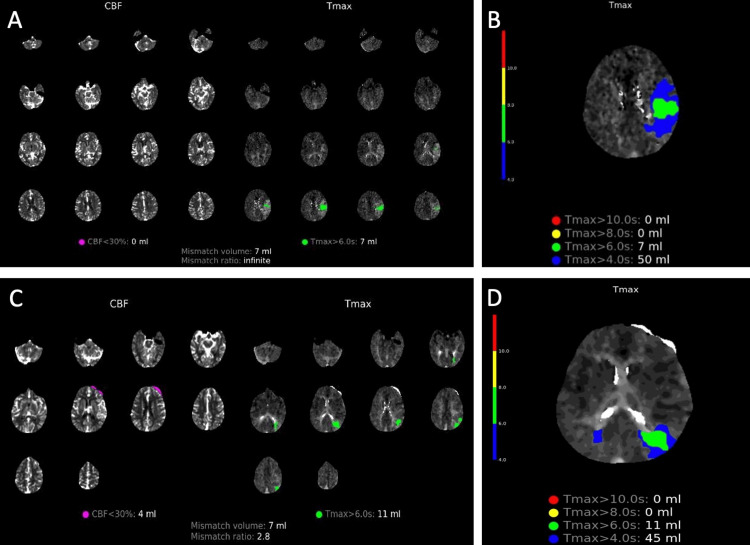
CT perfusion images A) CT perfusion analysis depicting possible core and penumbra at initial presentation. B) Detailed image of CT perfusion at initial presentation with various Tmax values. C) CT perfusion analysis depicting possible core and penumbra on day 11 of admission. D) Detailed image of CT perfusion on day 11 with various Tmax values CT: computed tomography; CBF: cerebral blood flow; Tmax: time-to-maximum
